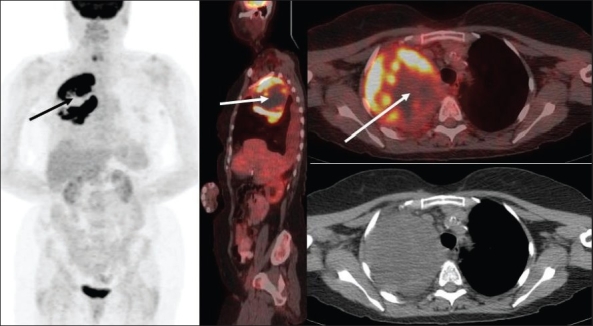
Axial fused PET/CT (top right) and CT (bottom right) images at the level of the upper lungs, with a sagittal fused PET/CT image (middle) and an MIP image (on left), from a PET study of a 71-year-old lady with a large right upper lobe mass. Bronchoscopic evaluation and two CT-guided biopsies failed to reveal malignancy. PET scan clearly shows a large malignant right upper lung mass with a large ‘cold’ central necrotic area (arrows) and intense FDG uptake peripherally. A third CT-guided biopsy from the periphery of the mass based on the PET findings confirmed NSCLC
